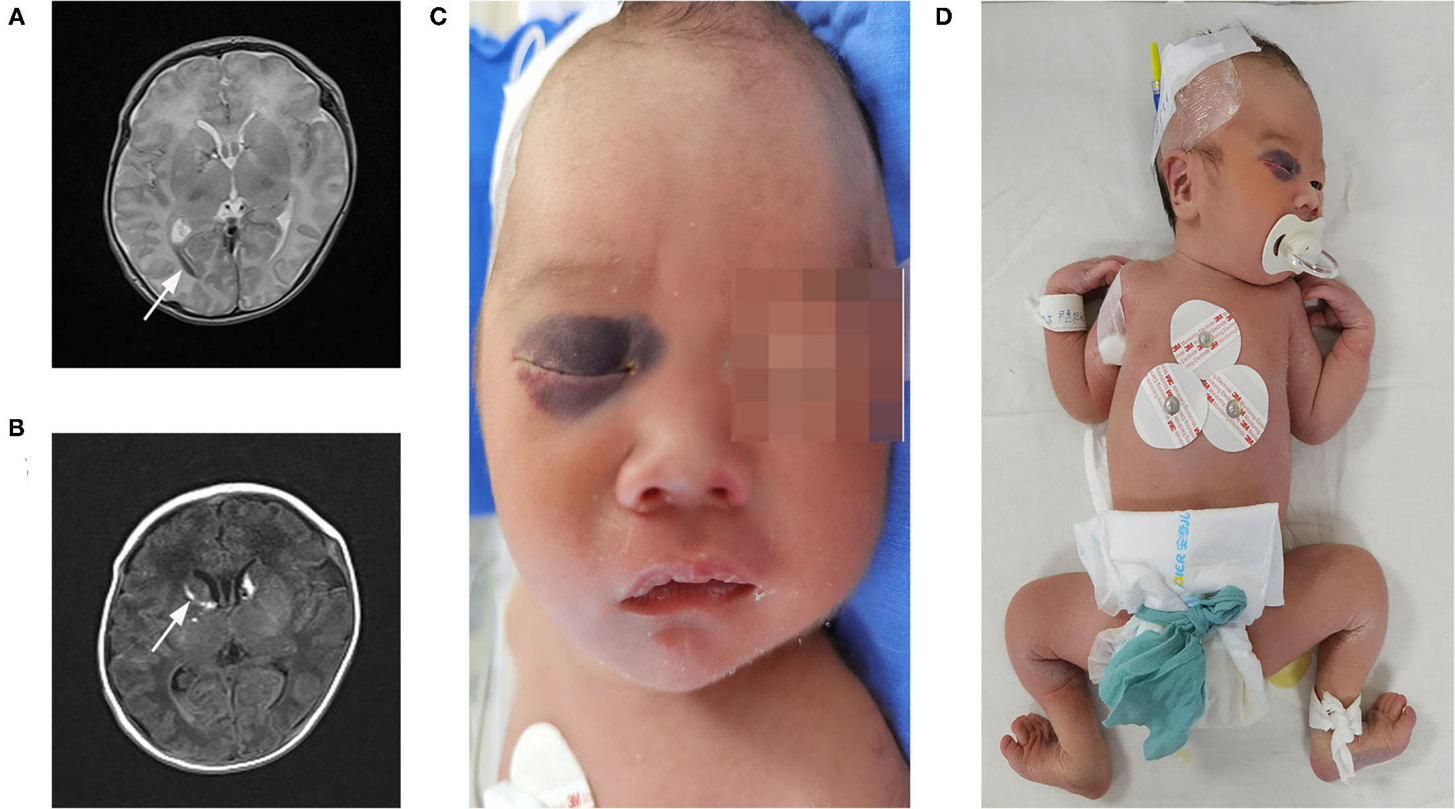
Clinical features and imaging manifestations of proband. (A,B) Cranial MRI: intraventricular hemorrhage and subarachnoid hemorrhage (direction of arrow). (C) Facial bruises. (D) Wrist, excessive abduction of the bilateral hip joints, bipedal valgus, and excessive flexion.
